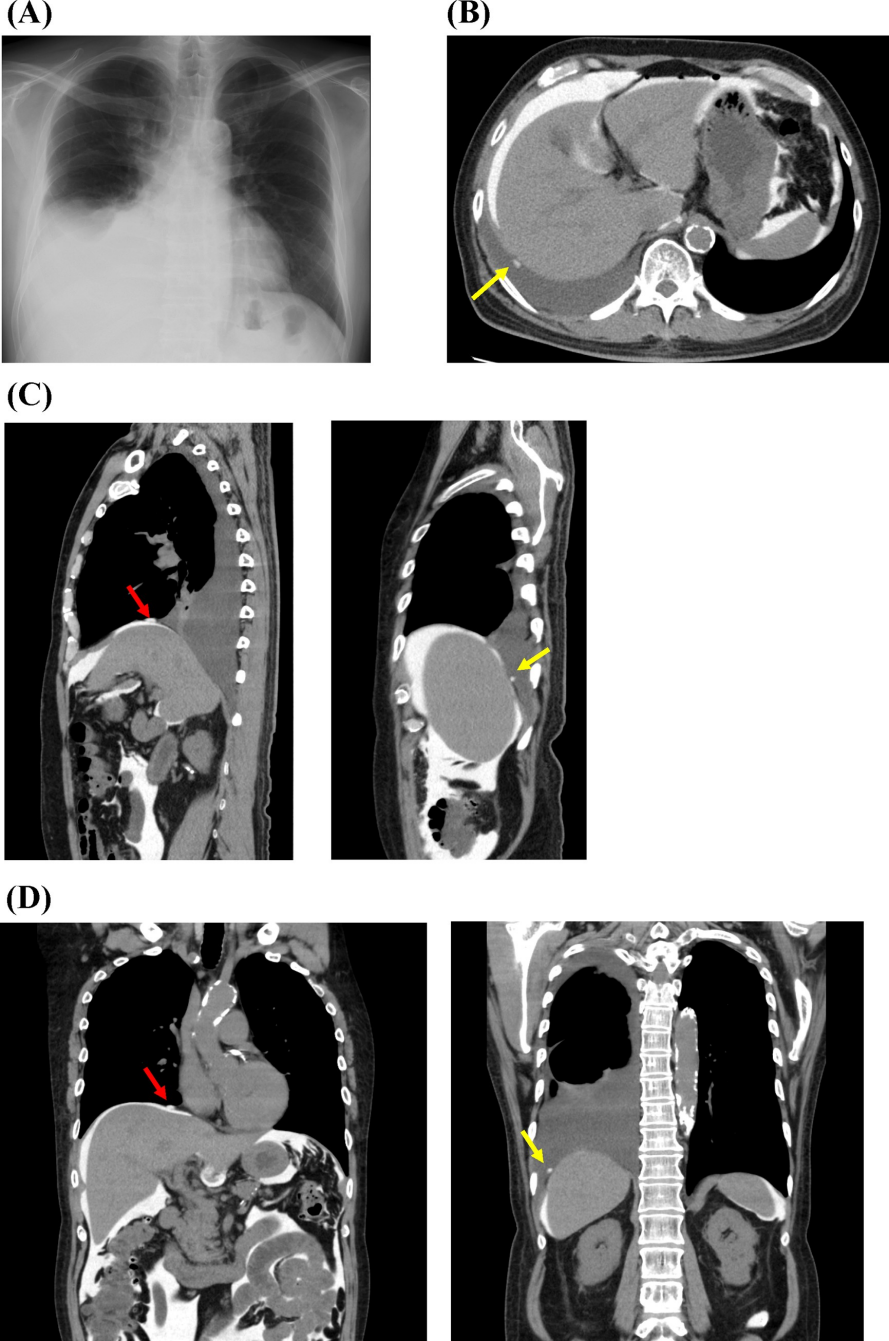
A case of hydrothorax in a peritoneal dialysis patient with two possible penetrated sites. (A) Chest x-ray at the time of hydrothorax diagnosis. (B, C, D) Penetrated sites on the diaphragm as revealed by CT peritoneography on the transverse, transverse, sagittal, and coronal view, respectively (red and yellow arrows are penetrated sites).
